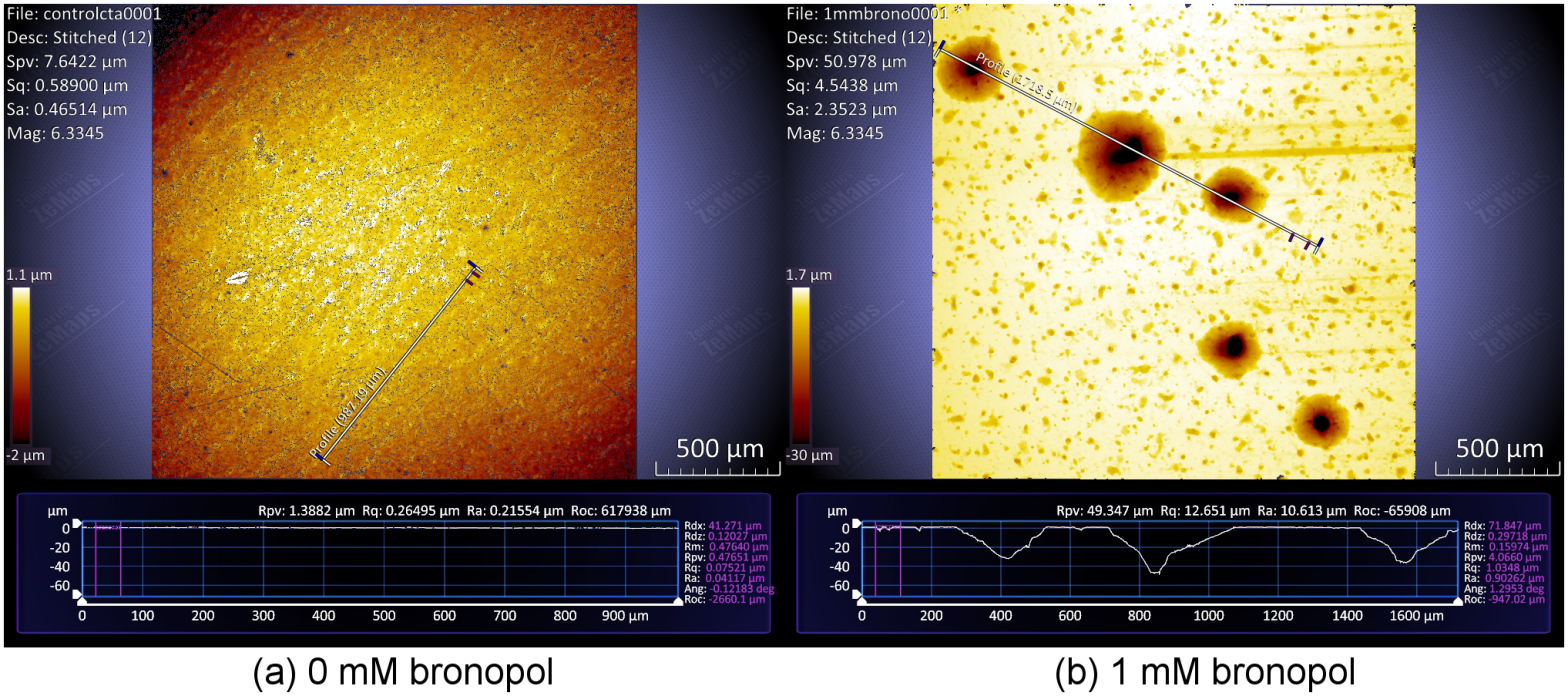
Optical profilometer image and pit depth profile for CT coupon in the (a) absence and (b) presence of bronopol (1 mM) after one week of incubation with N_2_-CO_2_ headspace.
